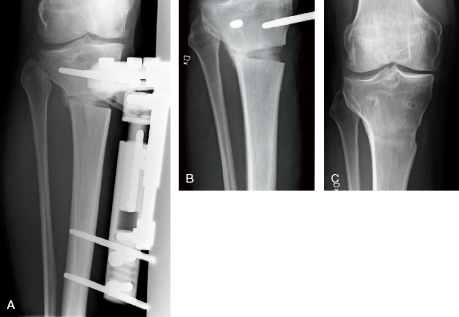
Hemicallotasis osteotomy using the Orthofix T-garche as external fixator. A. Successive lengthening takes place. The HKA angle is slowly normalized and the frame is locked at the desired angle. The frame is kept until bone healing. B. When sufficient callus appears to be present by ultrasound and radiography, the frame is removed temporarily. The patient is allowed to bear weight before the final decision to remove the frame. This decision is made blind regarding the pharmacological treatment. C. Radiograph showing a healed osteotomy at 1.5 years.
